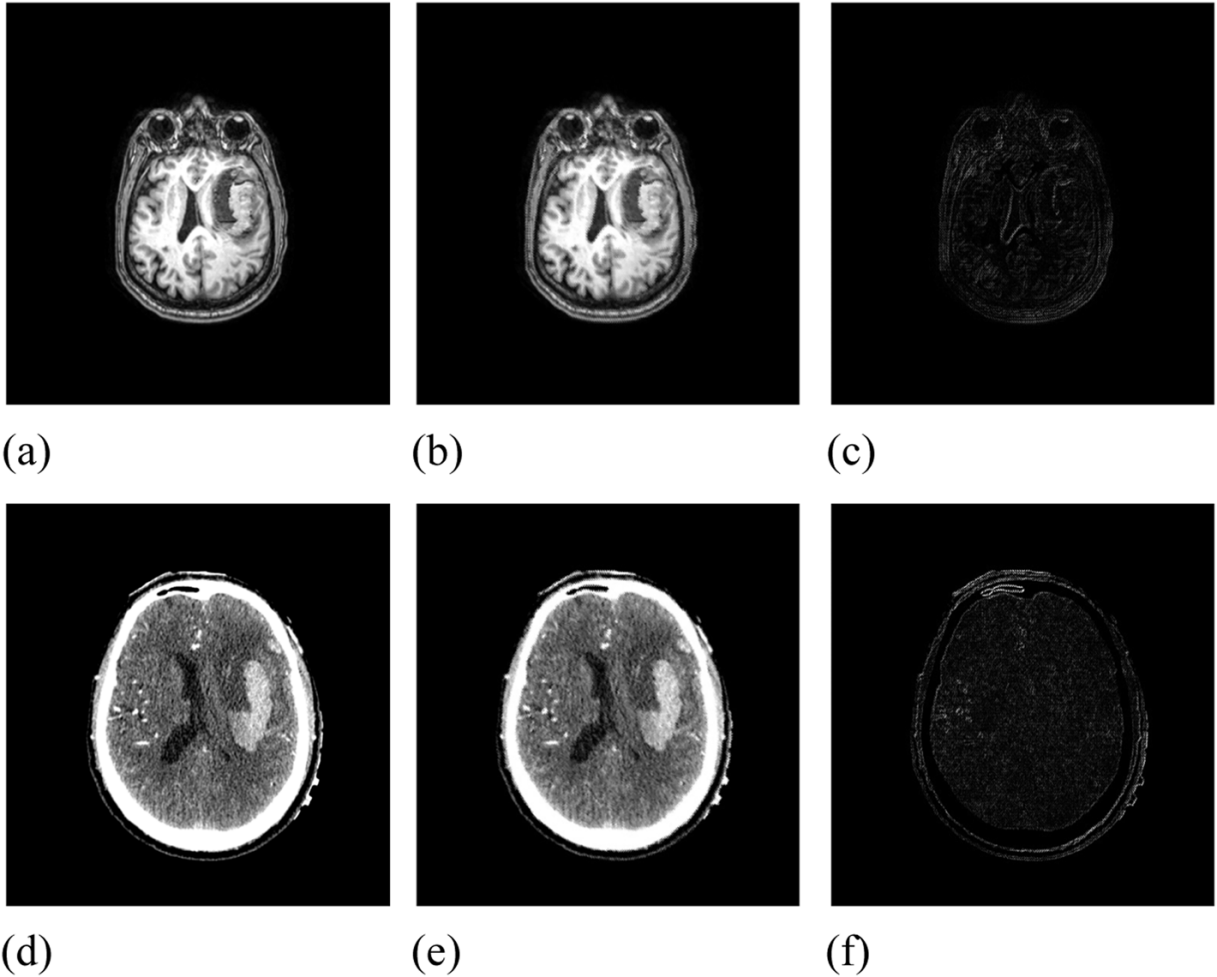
Initial image data. (a): MRI reference image; (b): MRI moving image; (c): Difference between MRI reference image and moving image; (d): CT reference image; (e): CT moving image; (f): Difference between CT reference image and moving image
